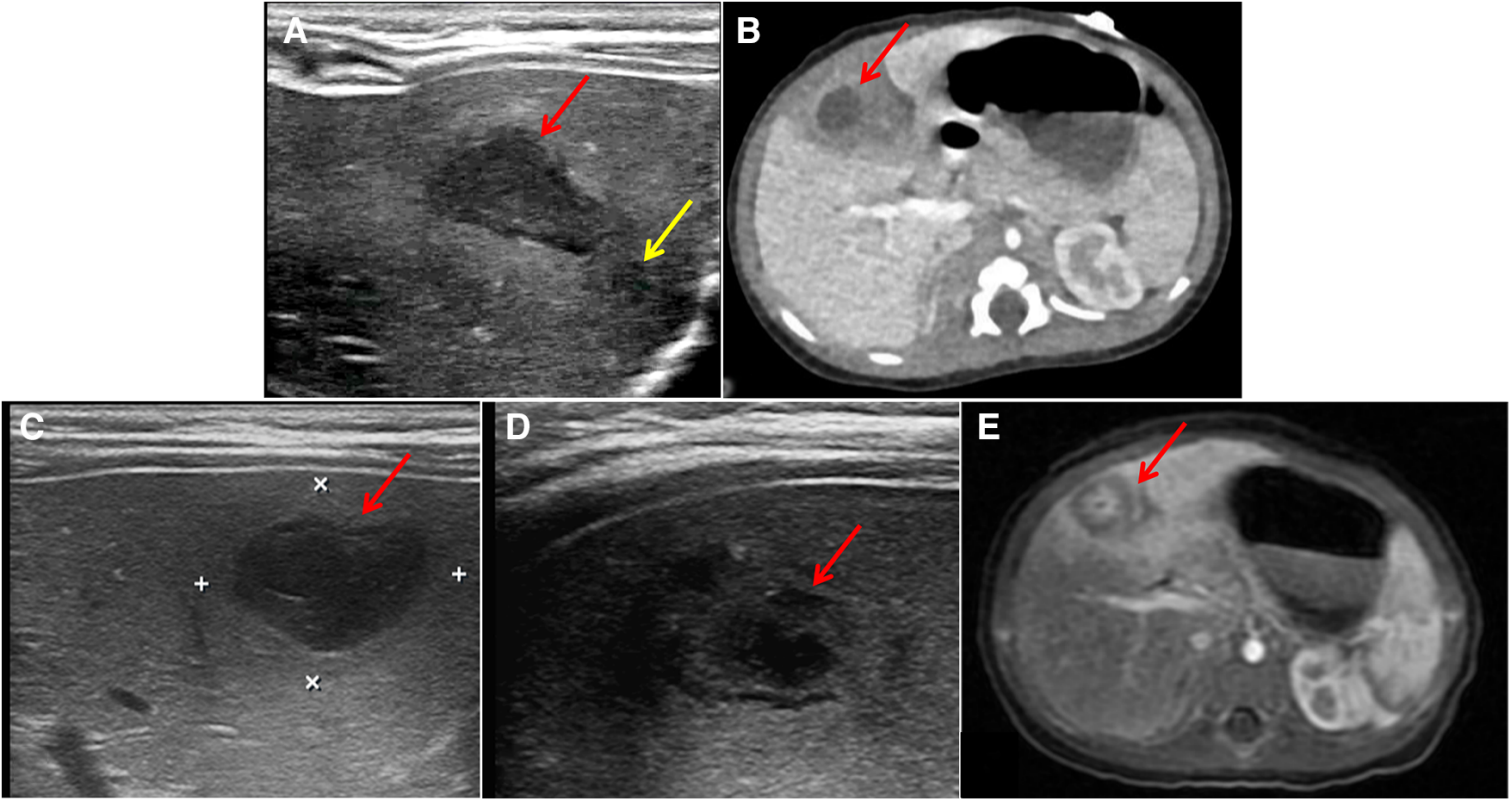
Imaging examination upon admission and reexamination of imaging examination. (A) Abdominal ultrasound: Irregular hypoechoic area in the S4 segment of the left lobe of the liver (Red arrow, 21 mm × 10 mm); A low echo mass (Yellow arrow, 5 mm × 5 mm) can be seen next to it, there was no obvious blood flow signal. (B) Abdominal CT plain scan and enhancement: liver S4 segment abscess (Red arrow, 29 mm × 36 mm × 33 mm), circular low density non enhanced liquid density shadow with a size of approximately 12 mm × 8 mm can be seen inside it. (C,D) Recheck liver ultrasound on the 3rd day and 4th day of admission: no significant change in abscess size (Red arrow, 19 mm × 14 mm, 6 mm × 5 mm) and no new abscess. (E) Abdominal MRI plain scan and enhancement on the 21st day of admission: significant absorption of liver S4 segment abscess lesions, with local small pus cavities remaining (Red arrow, 15 mm × 11 mm × 19 mm).
